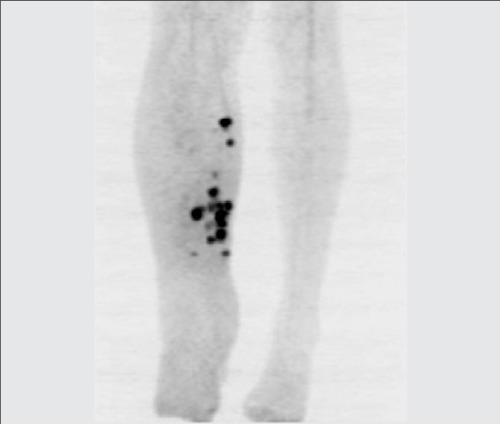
Maximum intensity projection (MIP) image of lowerextremity showed multiple increased activity (SUV_max_: 10.8) in thesubcutaneous tissue
